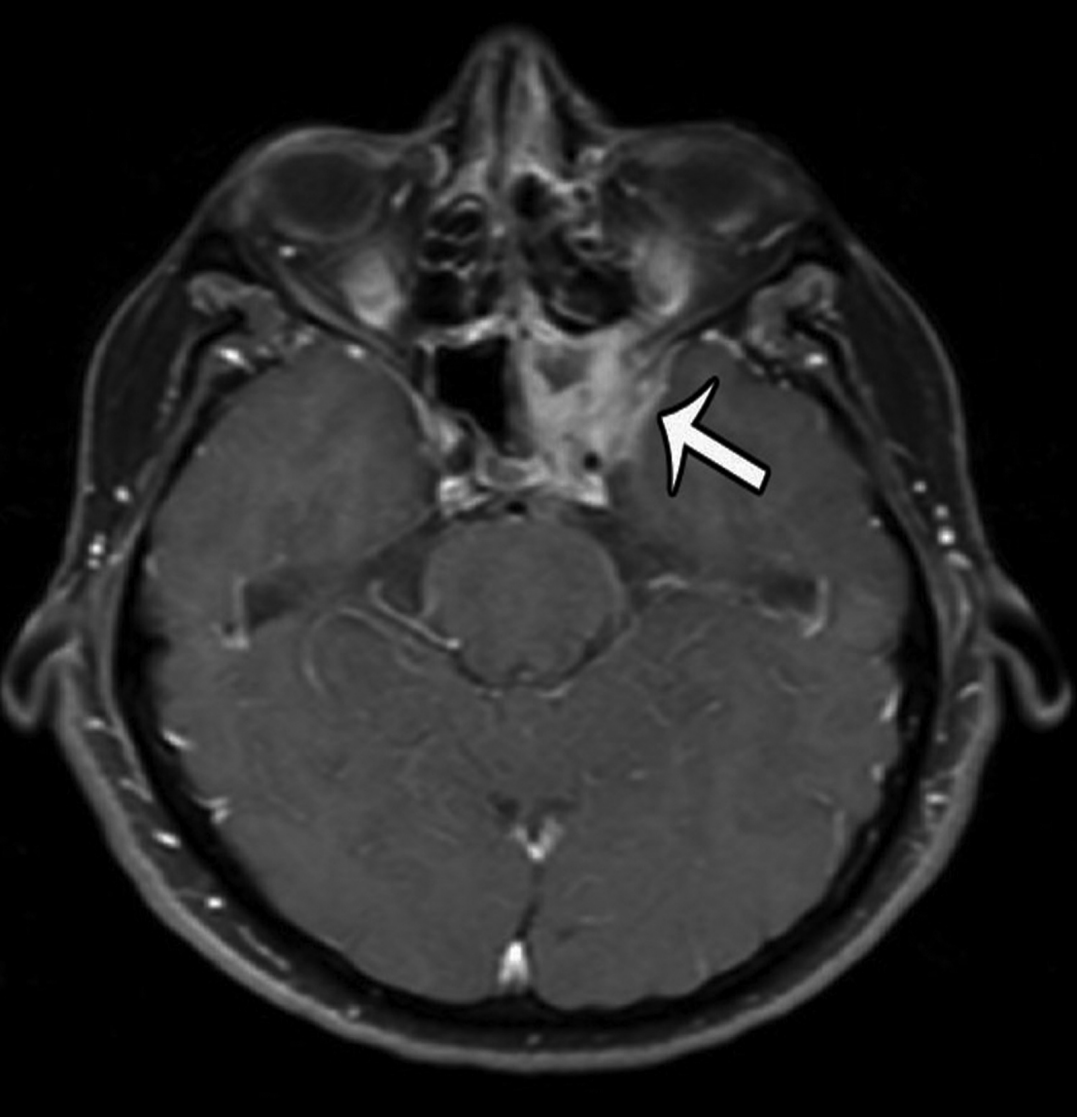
Pre-Treatment MRI Axial T1-weighted, contrast-enhanced image showing the same lesion as in Figure 1.
